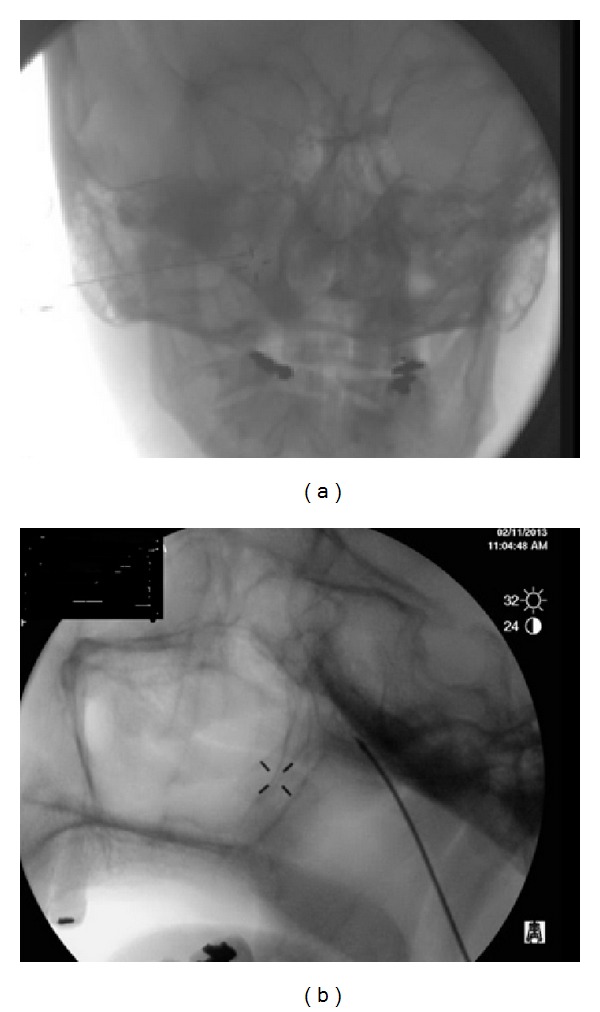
Anteroposterior view X-ray (a) and lateral X-ray (b) show the final radiofrequency needle position at the sphenopalatine fossa.
